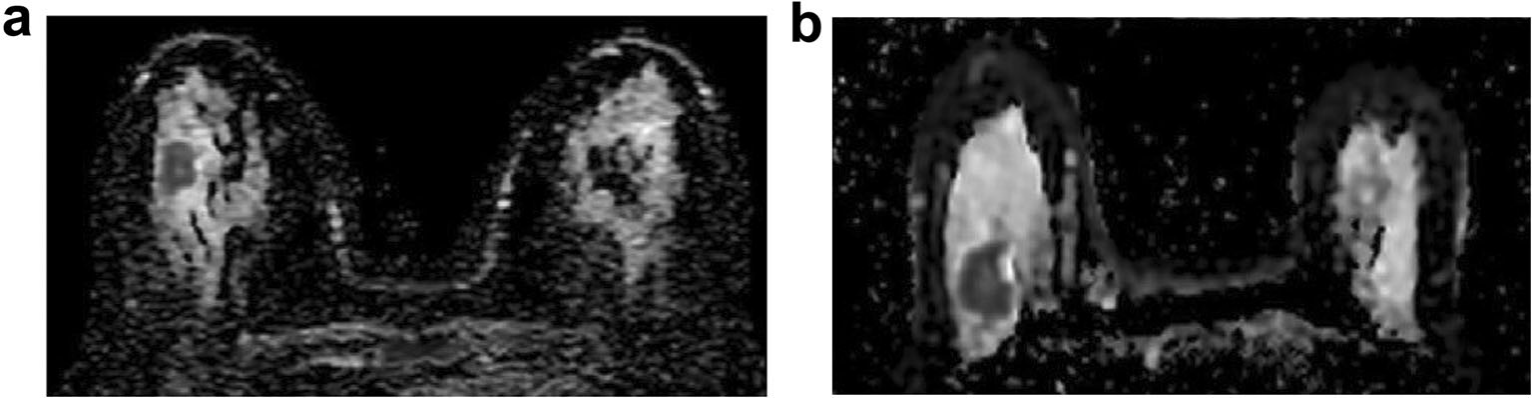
a ADC map of a 49-year-old patient with a luminal A cancer in the right breast. b ADC map of a 67-year-old patient with a luminal B cancer in the right breast. In our patient collective, radiomic signatures derived from DWI differentiated luminal A from luminal B cancers with an accuracy of 91.5 % when tumor segmentation was performed on the ADC map (89.5 % when segmented on high b-value DWI and copied to the ADC map). (DWI, diffusion-weighted imaging; ADC, apparent diffusion coefficient).
